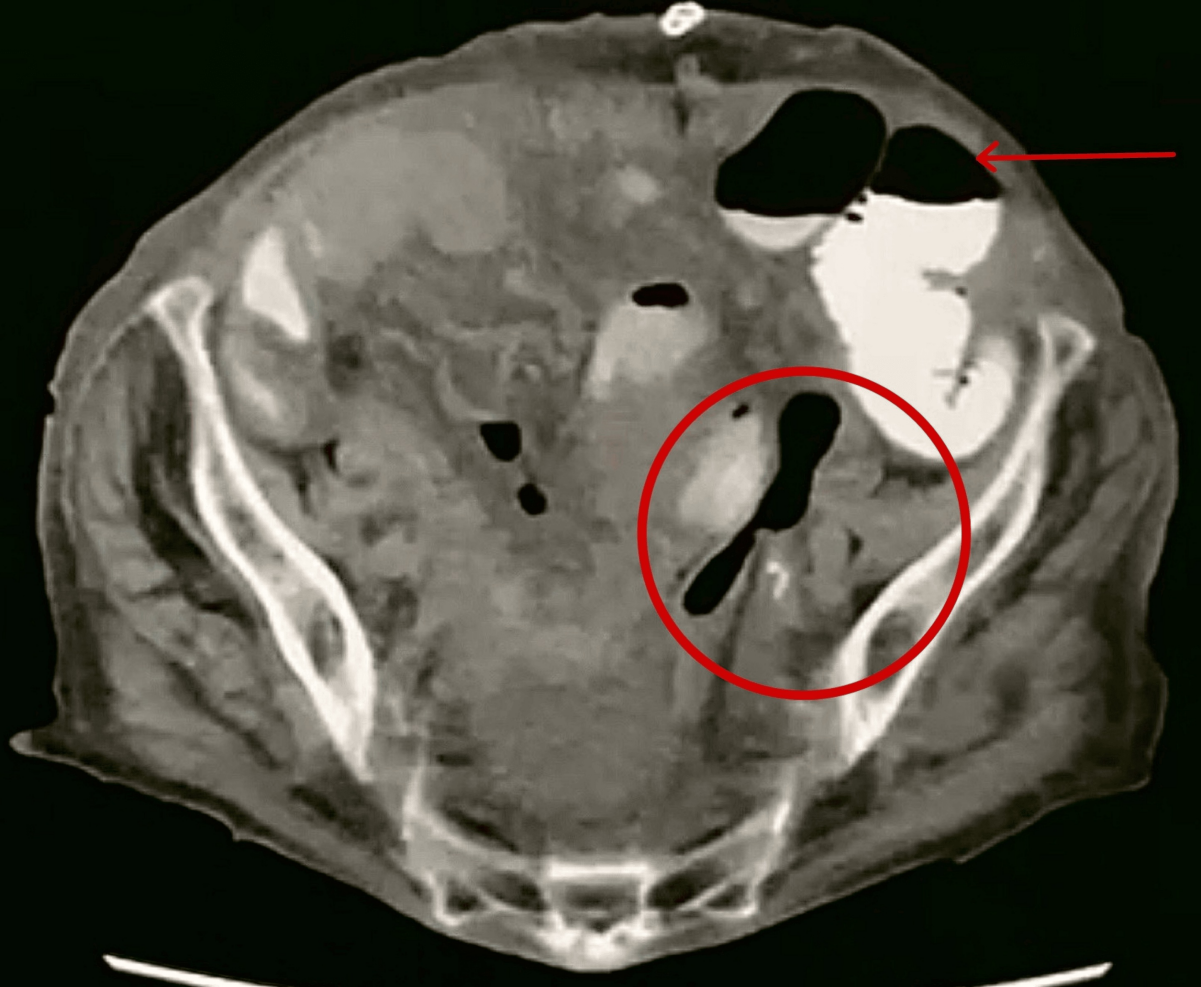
Axial CT with oral contrast demonstrating air-fluid levels (arrow) and incomplete passage of contrast to the distal bowel (circle)
